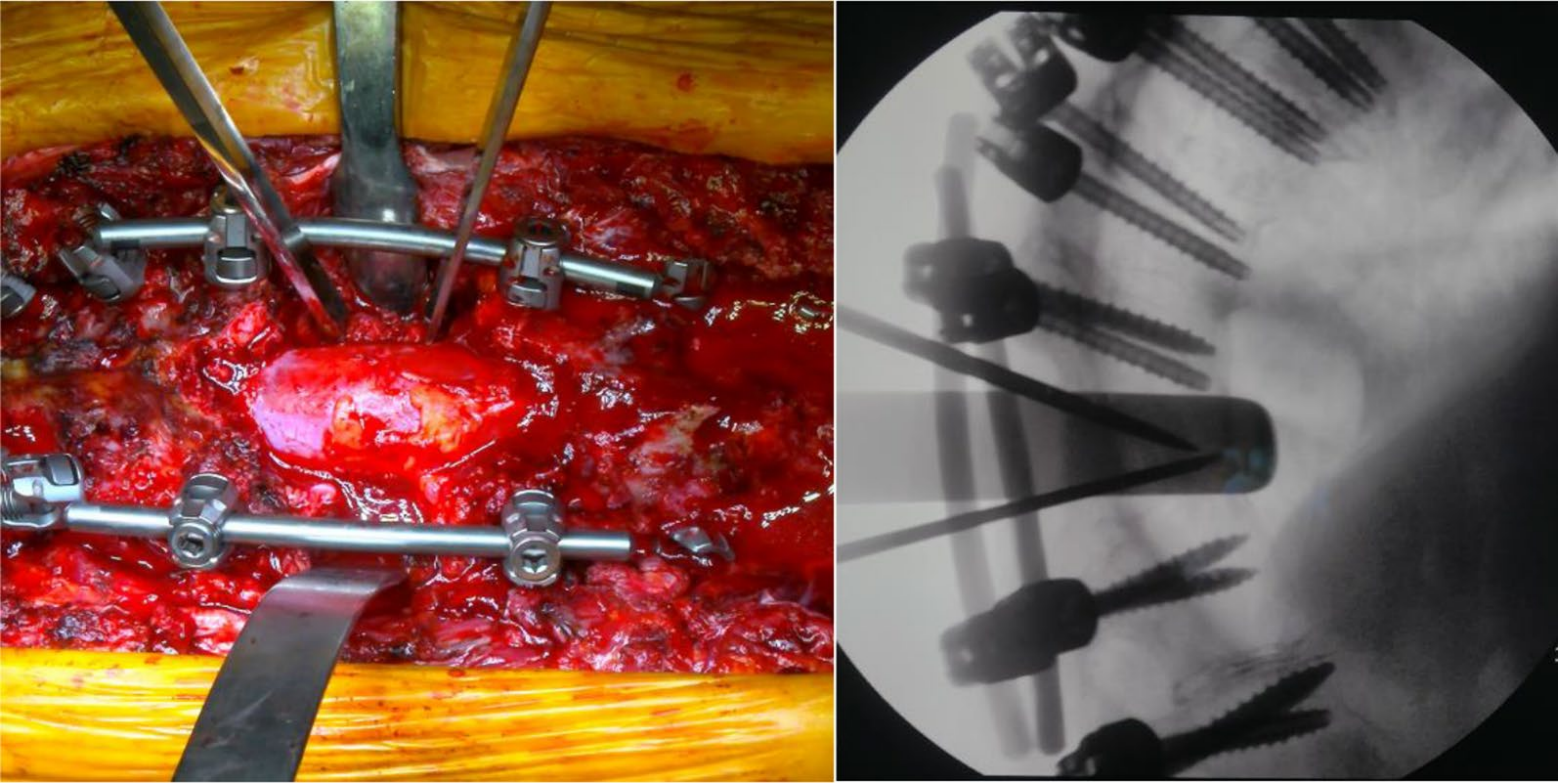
Surgical and radiographic pictures of the first PSO, performed at T12 using a bone scalpel
